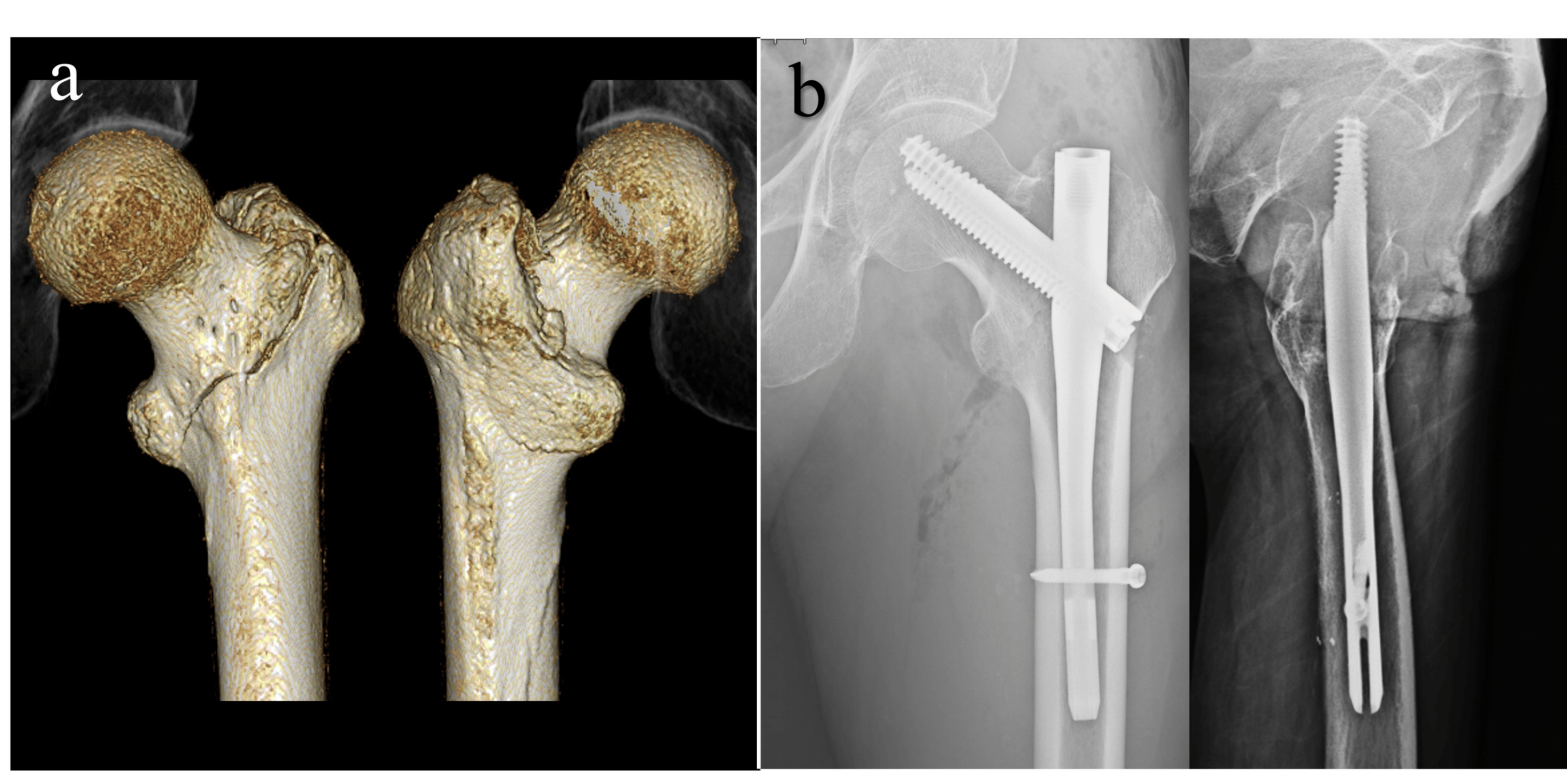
Pre- and postoperative imaging (a) Preoperative three-dimensional computed tomography (3D-CT) showing a nondisplaced intertrochanteric fracture of the left femur. (b) Postoperative plain radiographs after short intramedullary nailing
